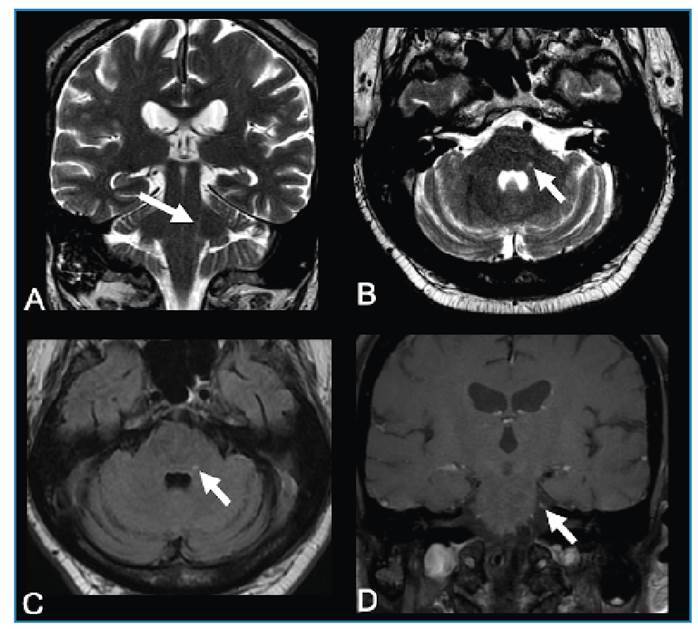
Trigeminal neuralgia due to varicella zoster virus reactivation. Brain MRI demonstrated a longitudinal lesion with hyperintense signal on T2-weighted imaging in the left spinal nucleus of the trigeminal nerve, in the coronal (arrow in A) and axial (arrow in B) planes and on an axial FLAIR (arrow in C), associated with gadolinium enhancement in the cisternal segment of the left trigeminal nerve (arrow in D). PCR of a cerebrospinal fluid sample revealed VZV positivity.
